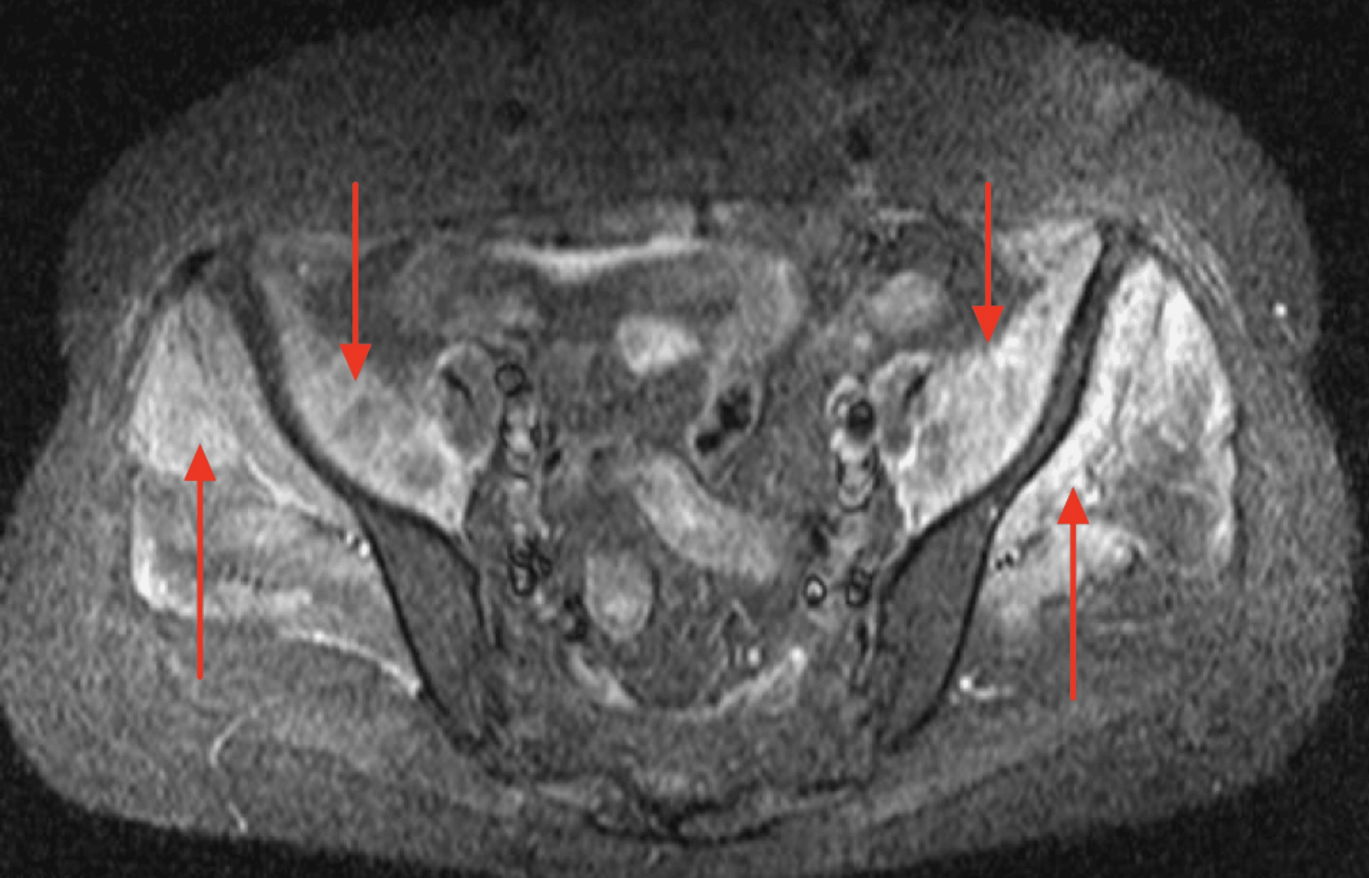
MRI of the Pelvic Muscles Revealing Edema Intense increased signaling in the iliacus, indicated by the red downward arrow, and gluteal muscles, indicated by the red upward pointing arrows, indicating edema within the muscles.
